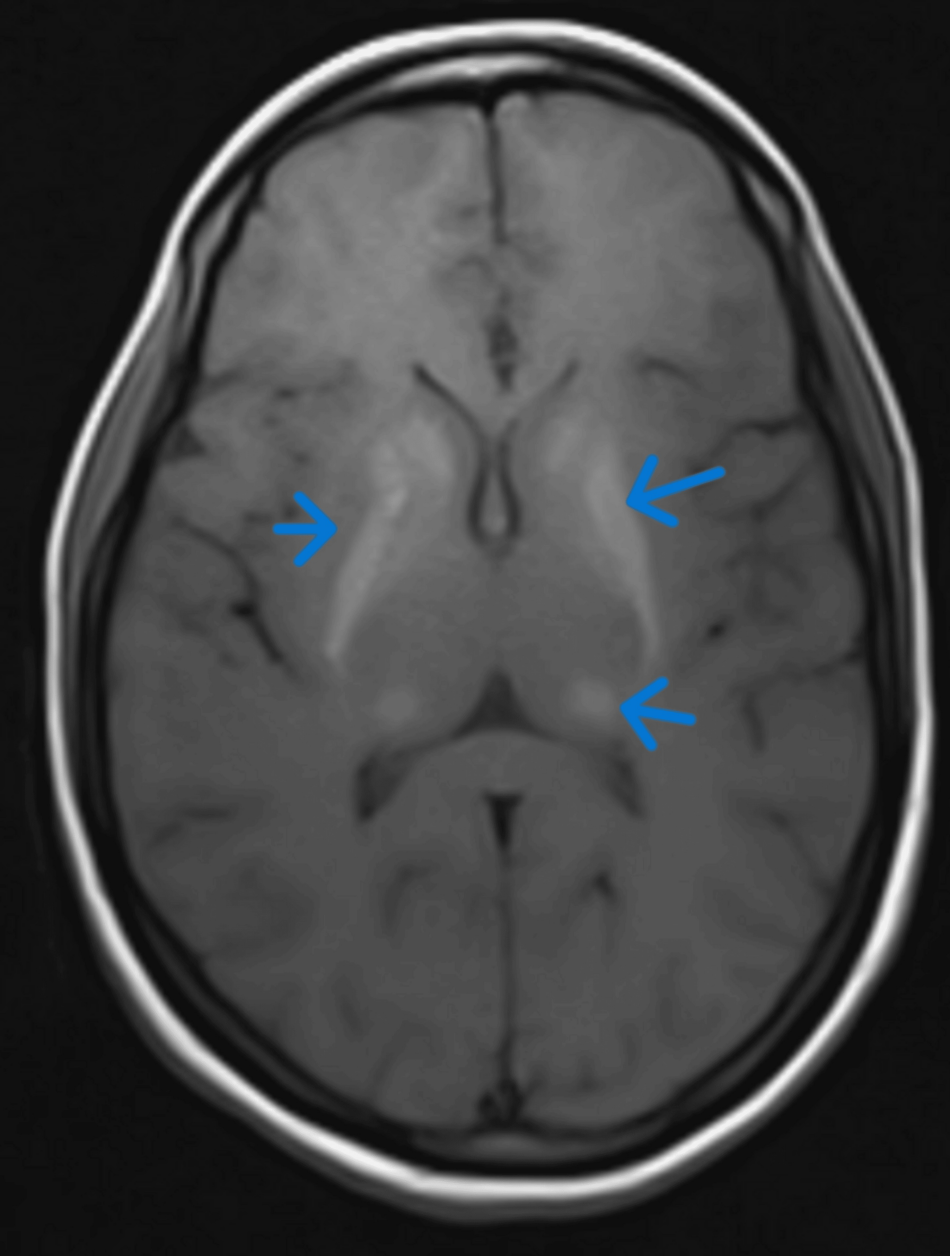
MRI brain T2 fluid-attenuated inversion recovery axial section showing hyperintensities in bilateral basal ganglia and thalamus. Blue arrow pointing toward hyperintense signal in basal ganglia and thalamus.
